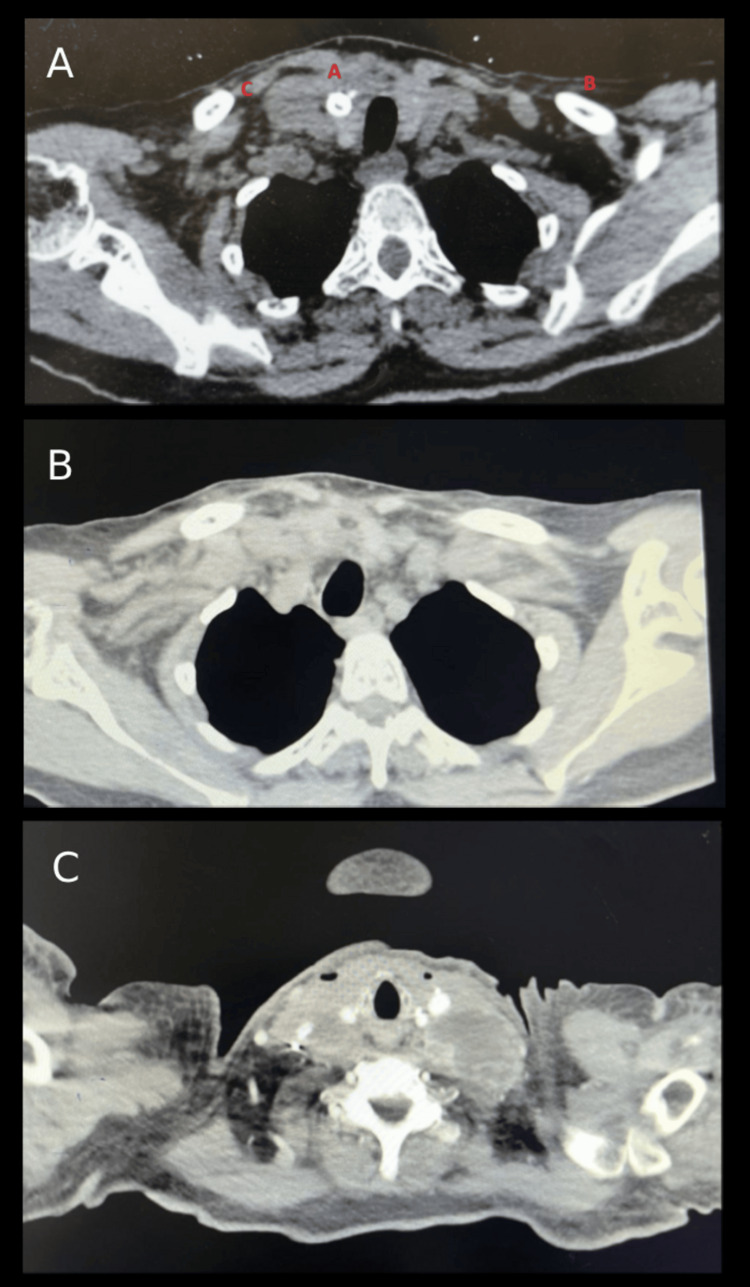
(A) Neck CT scan showing a heterogeneously enhanced thyroid gland with foci of calcification in the right lobe; (B) suspicious left supraclavicular lymph nodes, the largest measuring 13 mm x 13 mm; CT scan showing extension of thyroid into mediastinum; (C) thrombosed right internal jugular vein extending to superior vena cava; CT scan showing the postoperative hematoma.
